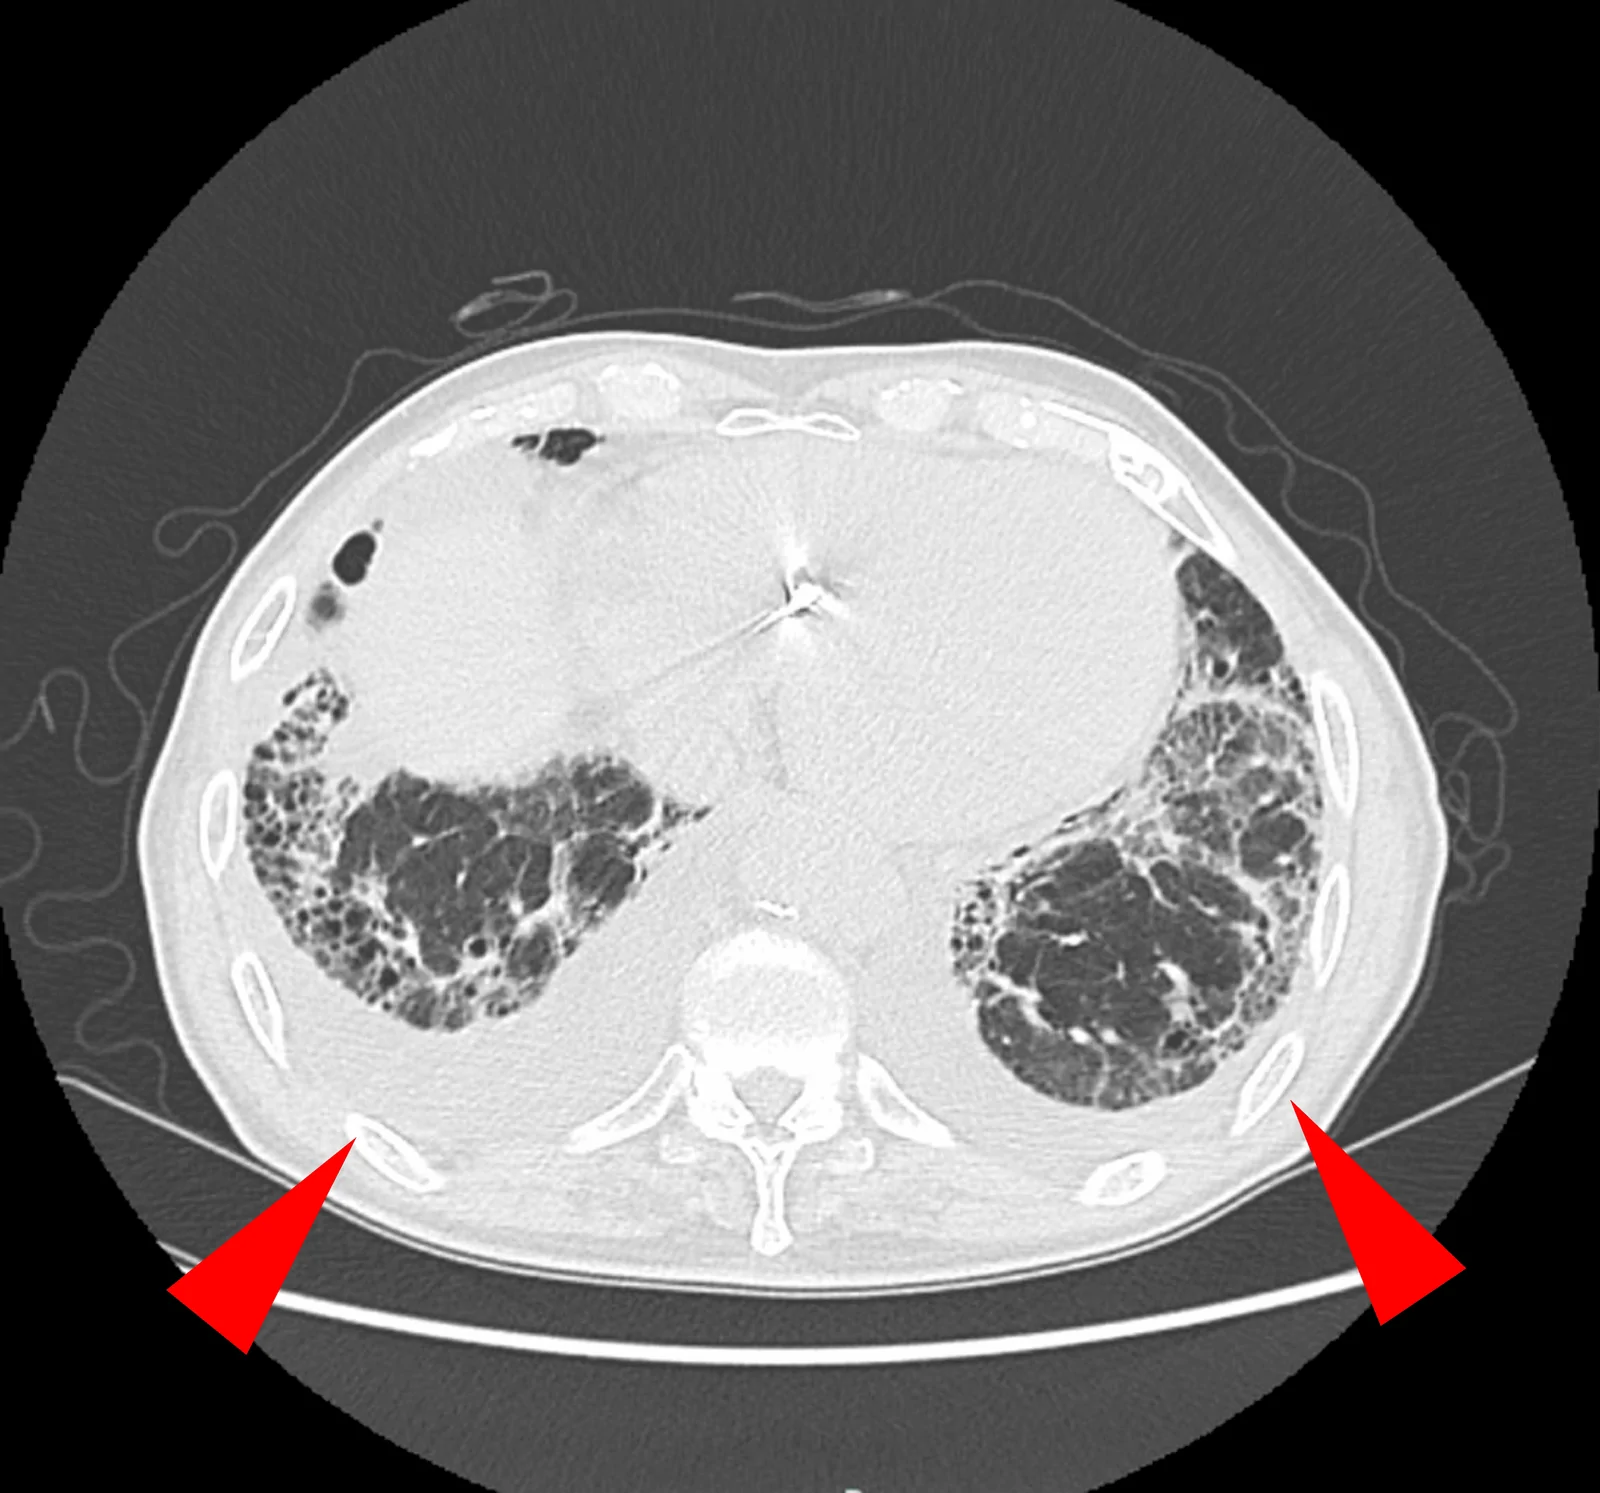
Chest computed tomography on hospital day 5 (one day before respiratory deterioration) Chest computed tomography demonstrating persistent bilateral ground-glass opacities and fibrotic changes with newly developed bilateral pleural effusions, highlighted by red arrowheads
